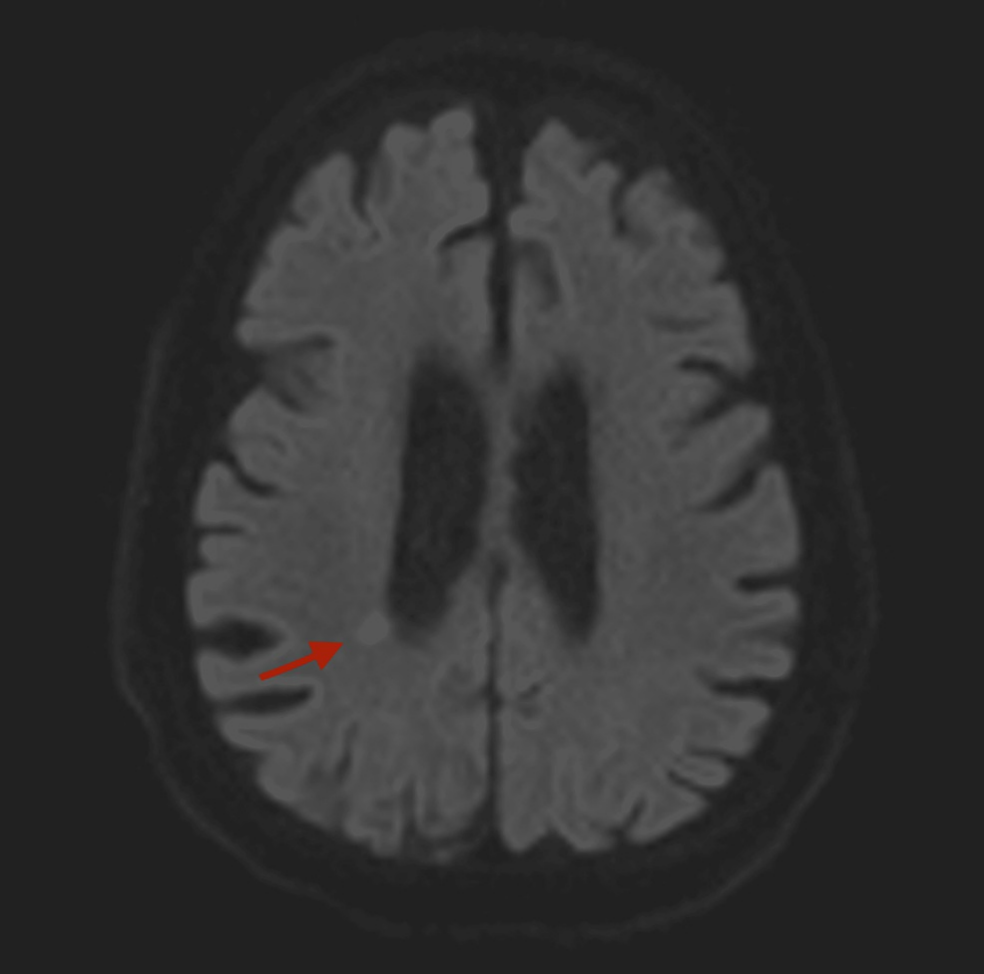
An axial section of magnetic resonance imaging of the brain, diffusion-weighted imaging (DWI) sequence, showing a small, restricted area in the posterior right periventricular partial region likely subacute infarction.
